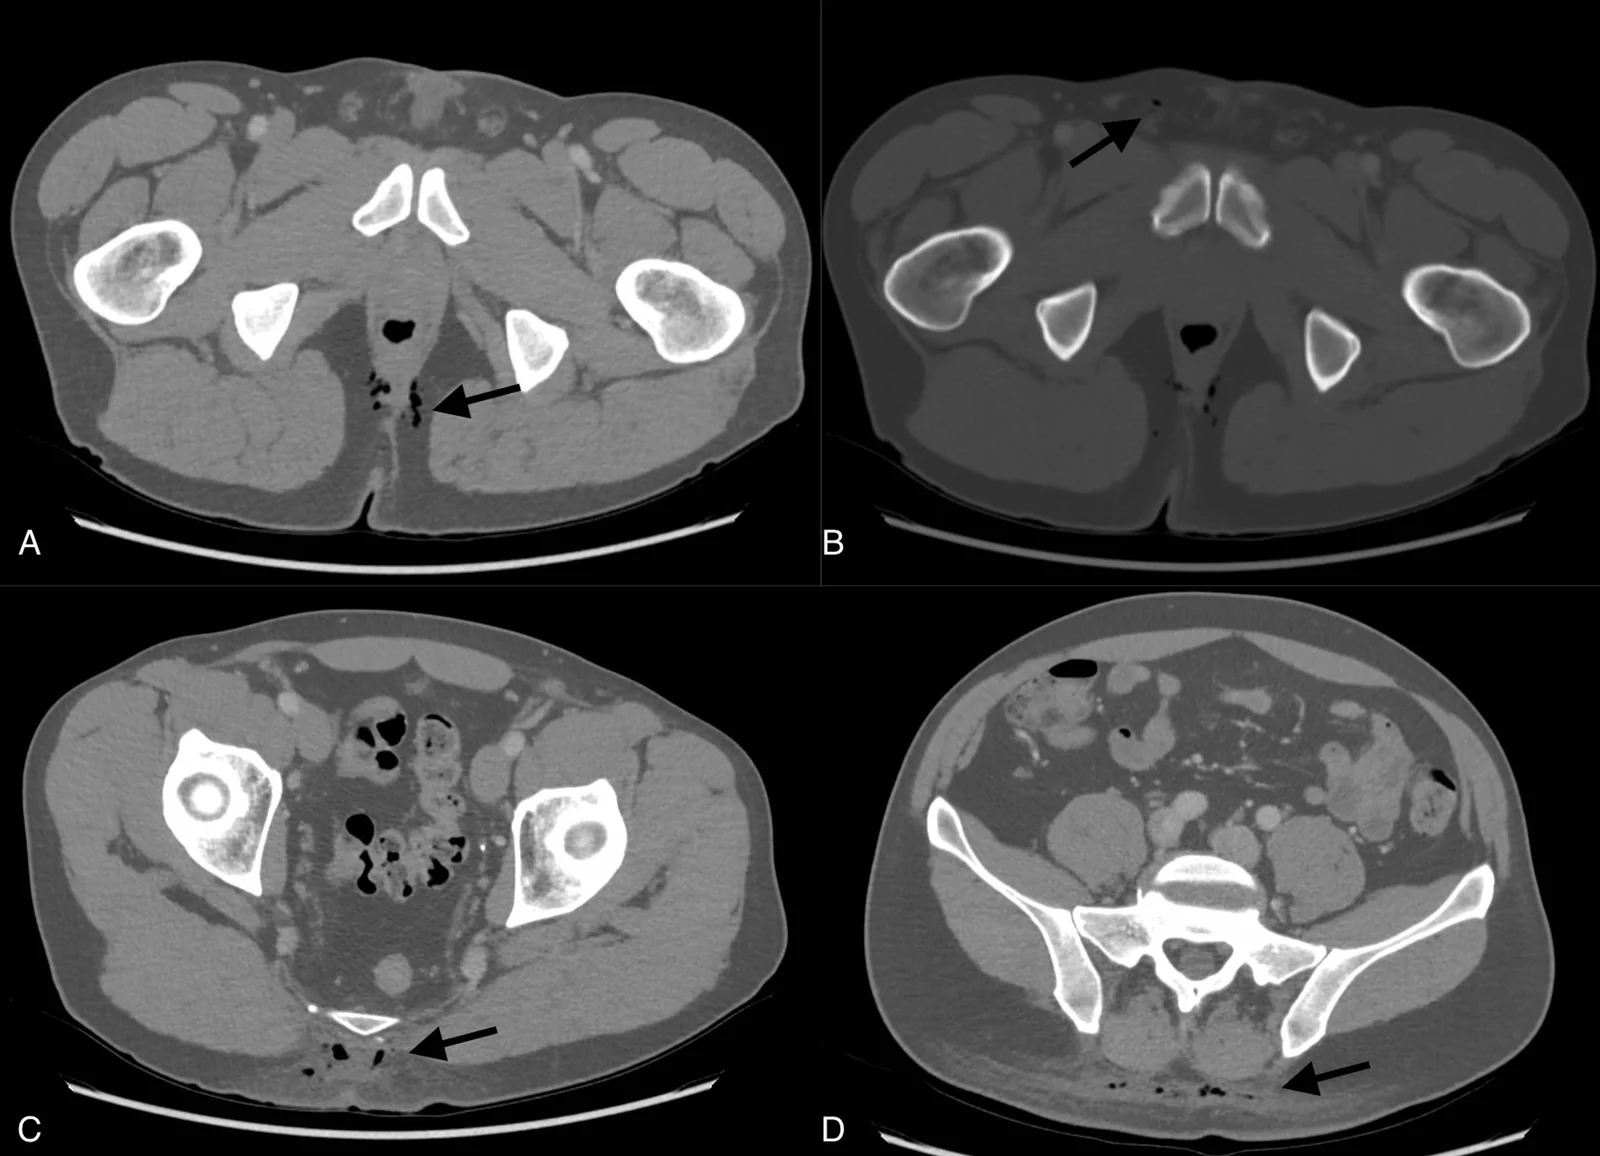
Axial view of multislice abdominal CT scan with intravenous contrast, showing subcutaneous air at the perirectum (a), right spermatic cord (b), coccyx (c), and sacrum (d).
